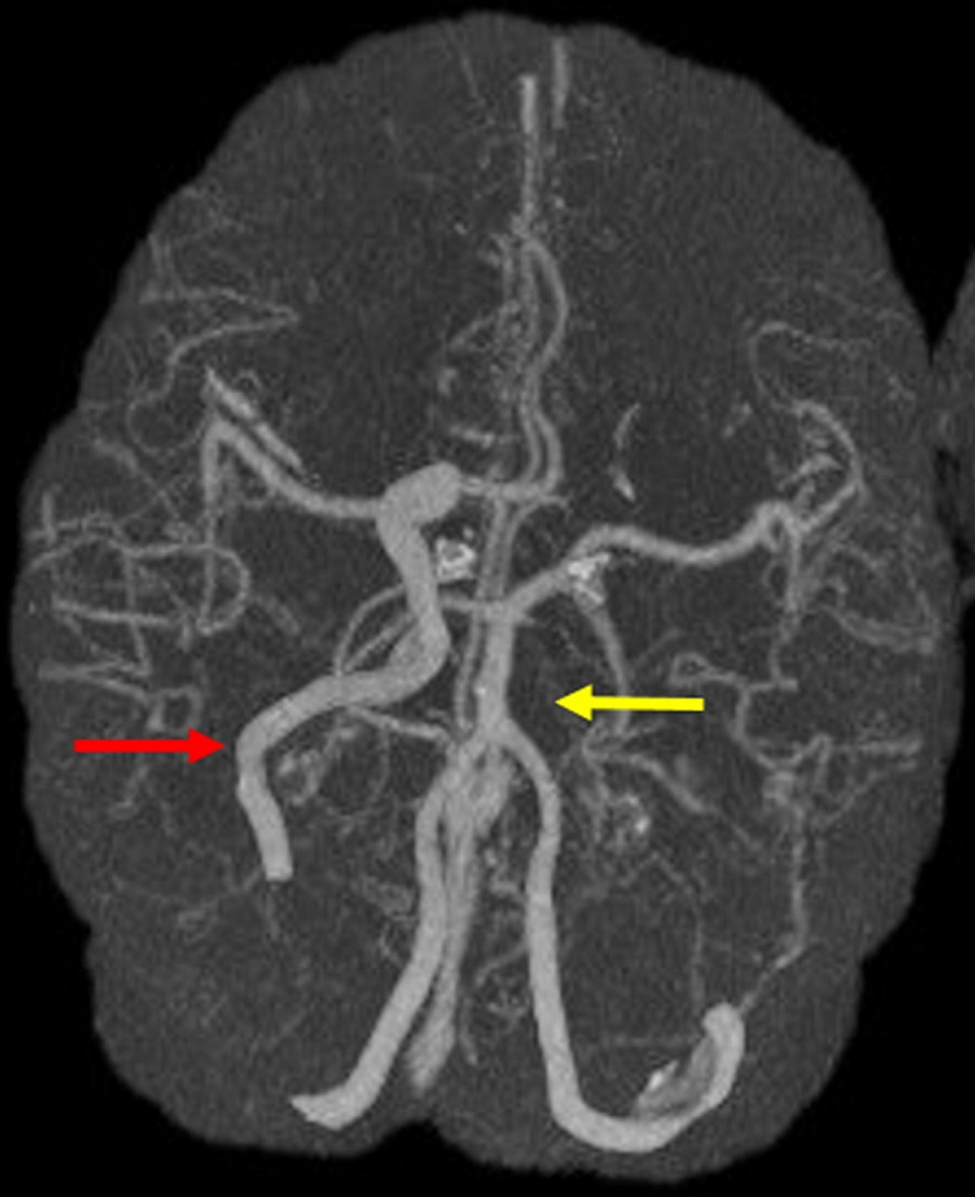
Computed Tomography Angiogram Showing Agenesis of the Left Internal Carotid Artery An axial maximum intensity projection computed tomography angiogram demonstrates agenesis of the left internal carotid artery (yellow arrow). The native right internal carotid artery is shown (red arrow).
